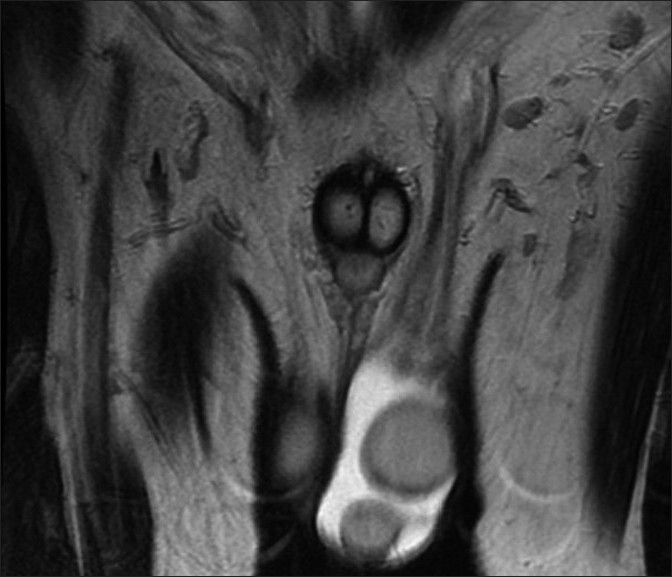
The presence of supernumerary testis that had the same signal characteristics as the normal testicles on MRI
